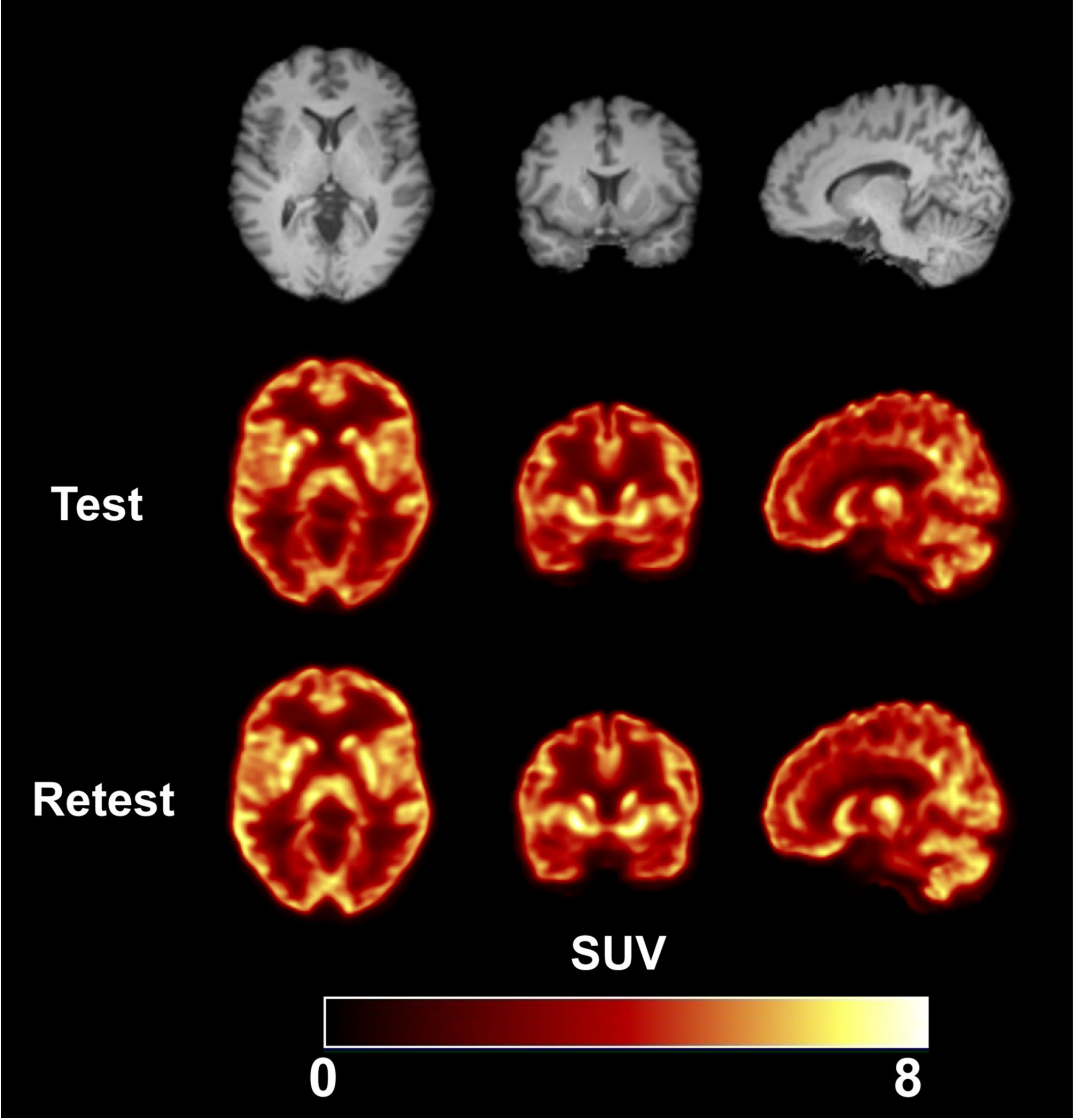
MR and co-registered PET SUV images (summed from 0–120 min of data) from test and retest scans in the same subject
